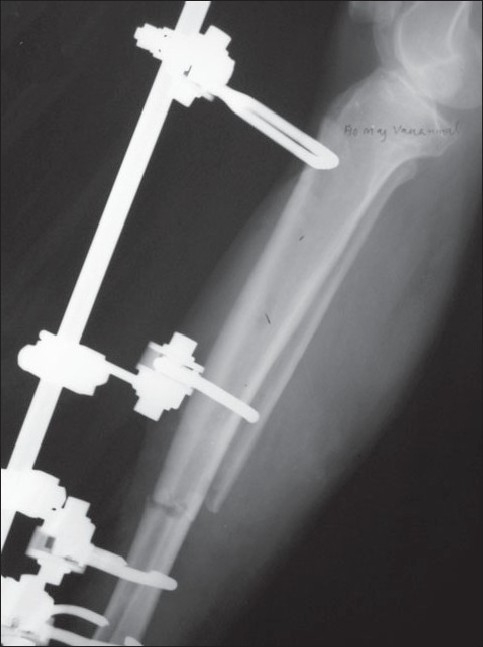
Plain radiograph of fracture of distal third tibia stabilized with pinless fixator. A well-aligned medullary canal with a four-clamp pinless frame can be seen.
